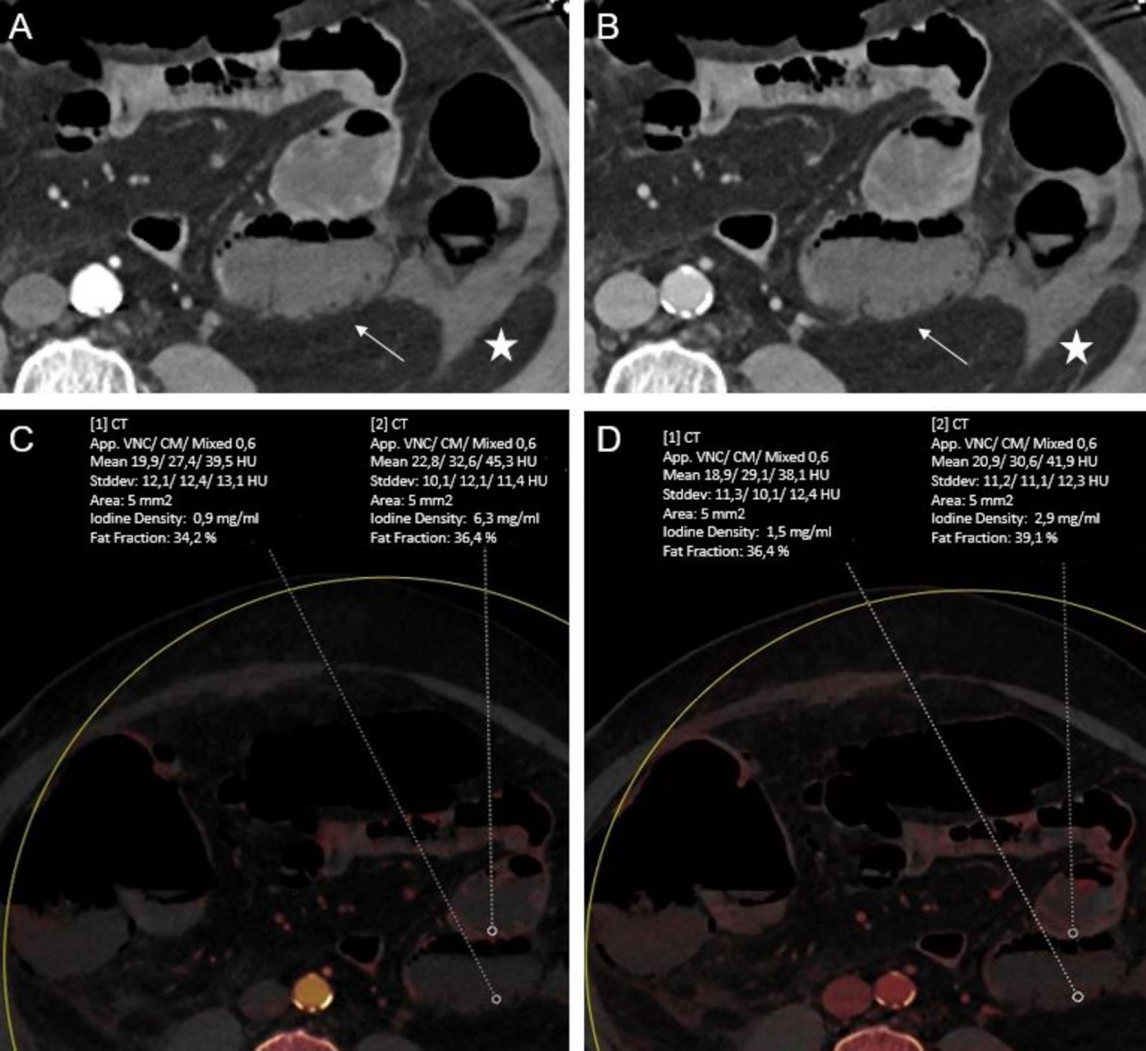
60-year-old woman with acute abdomen and elevated lactate. Conventional transversal late arterial (LA, A) and portal venous (PV, B) demonstrate free intraabdominal fluid (*star*) next to a small bowel loop (*arrow*). Corresponding dual-energy CT iodine maps based on LA and PV phase (C and D, respectively) demonstrate a greater difference in iodine concentration (IC) reduction of this small bowel loop compared with adjacent segments in the LA phase (LA: 0.9 vs 6.3 mg/ml; PV: 1.5 vs 2.9 mg/ml), indicating significant ischemia which was proven by surgery. CT value measurements showed only a slight reduction (LA: 39.5 vs 45.3; PV: 38.1 vs 41.9)
